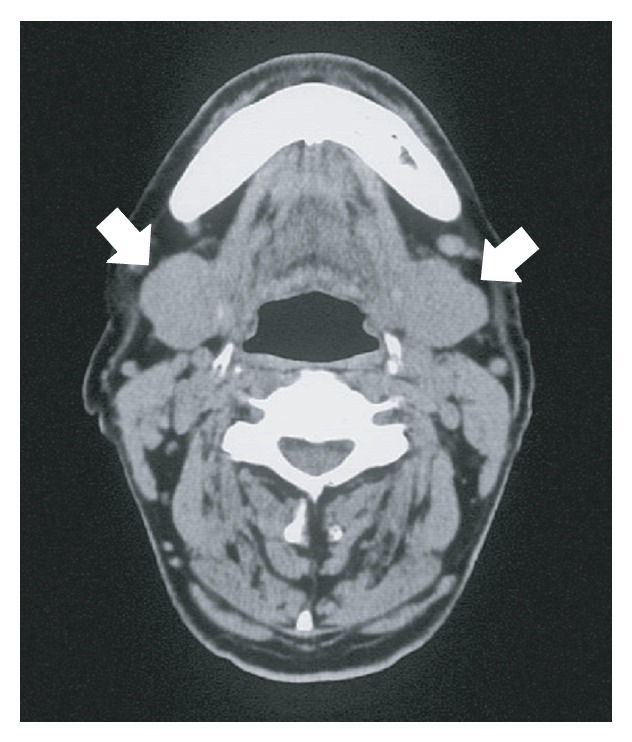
Cervical CT images of Case 1. Bilateral swelling of the submandibular glands (arrows).
